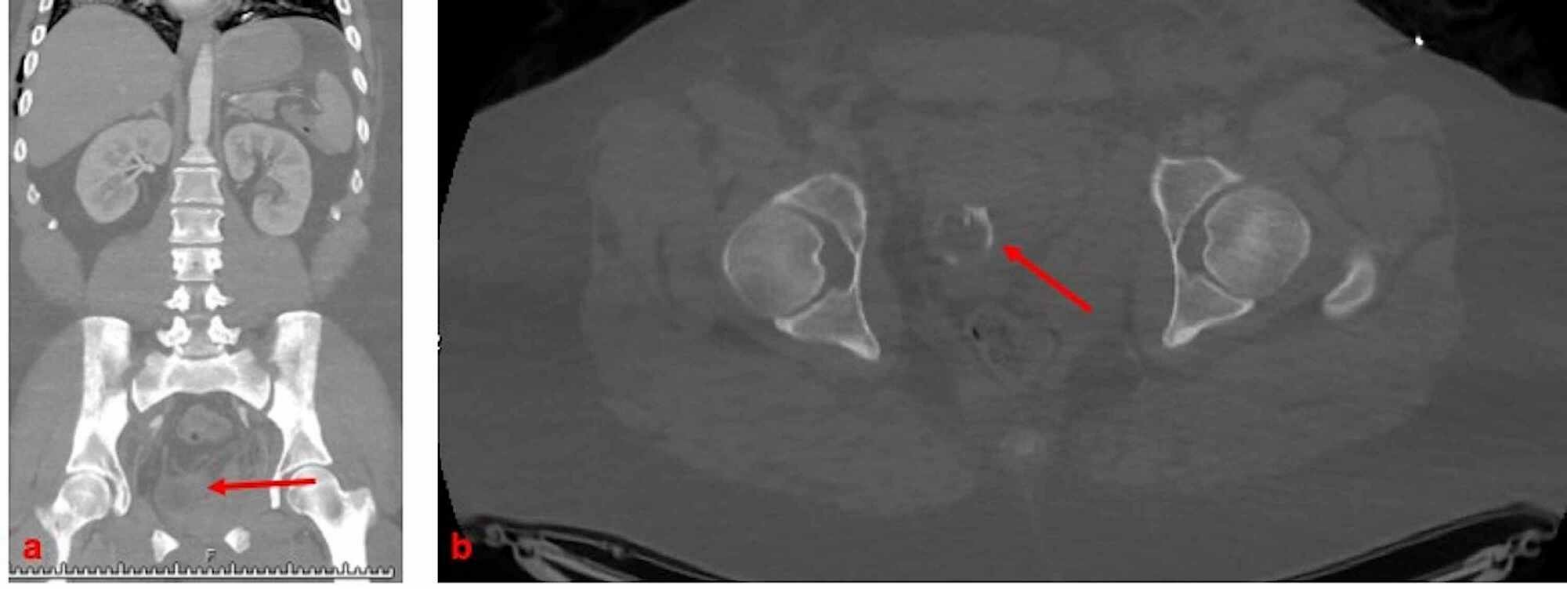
Preoperative CT scan demonstrating axial and coronal images of testicular dislocation adjacent to the pubis. CT: computed tomography
